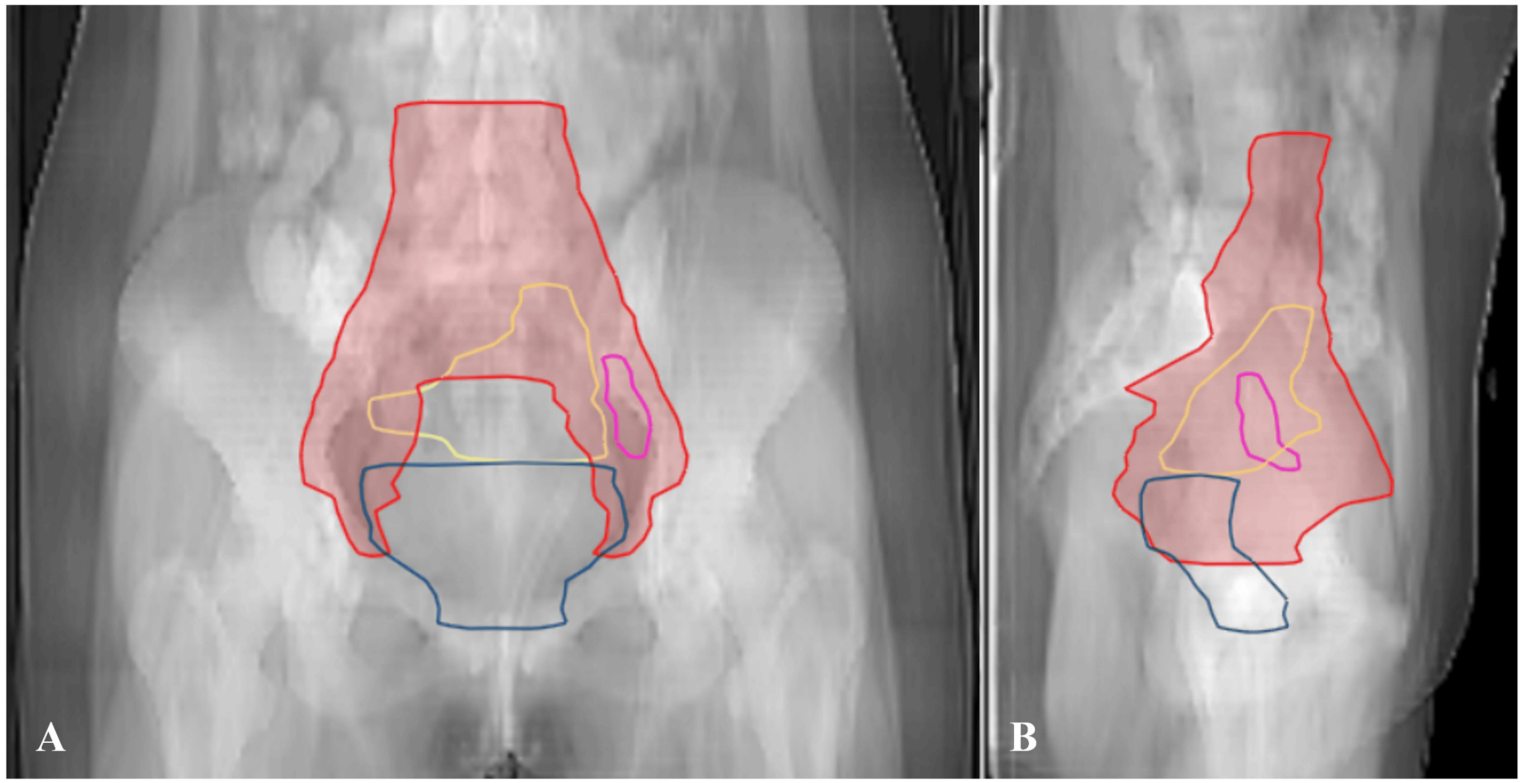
Example images of axial (A) and sagittal (B) slices of a planned CT scan from the patient. CTV-N is shown in red, CTV-U is shown in yellow, CTV-C is shown in blue, and GTVnd is shown in magenta. Abbreviations: CT, computed tomography; CTV-N, clinical target volume of the lymph; CTV-U, clinical target volume of the uterus; CTV-C, clinical target volume of the cervix; GTVnd, gross tumor volume node
